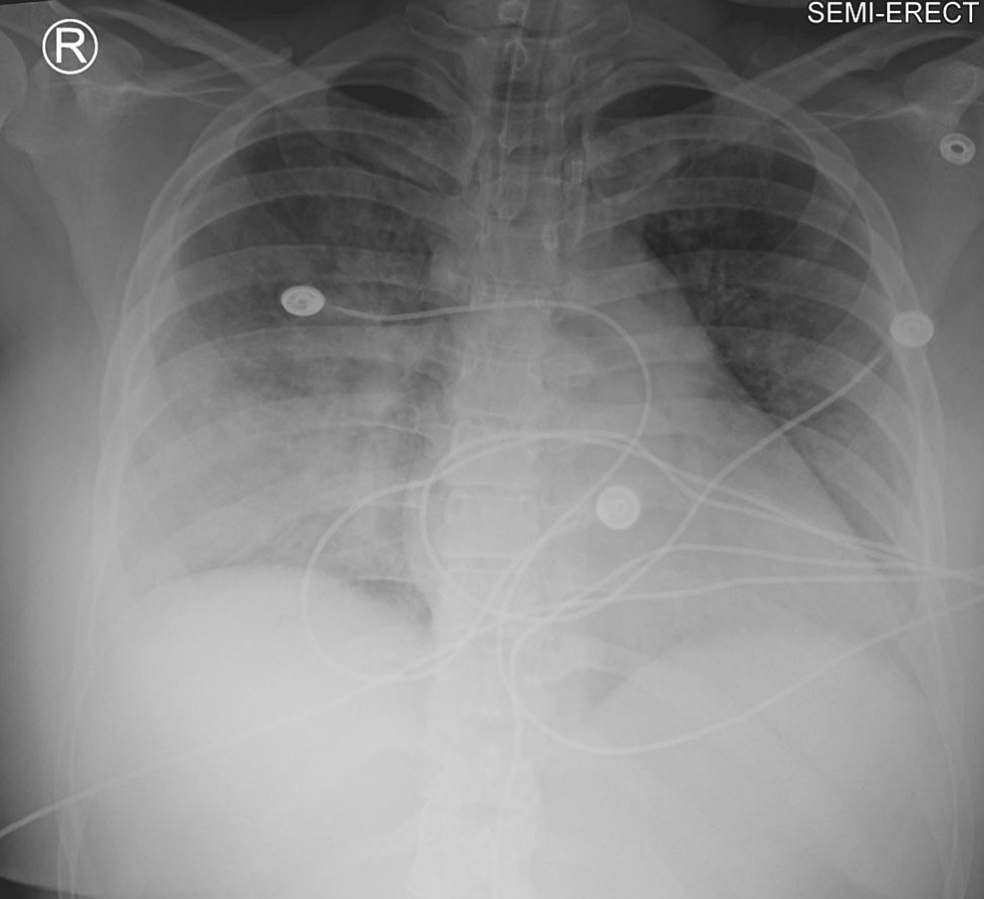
Chest X-ray obtained following diuresis. Redemonstration of diffuse pulmonary edema pattern with mild interval improvement compared to prior radiograph.
